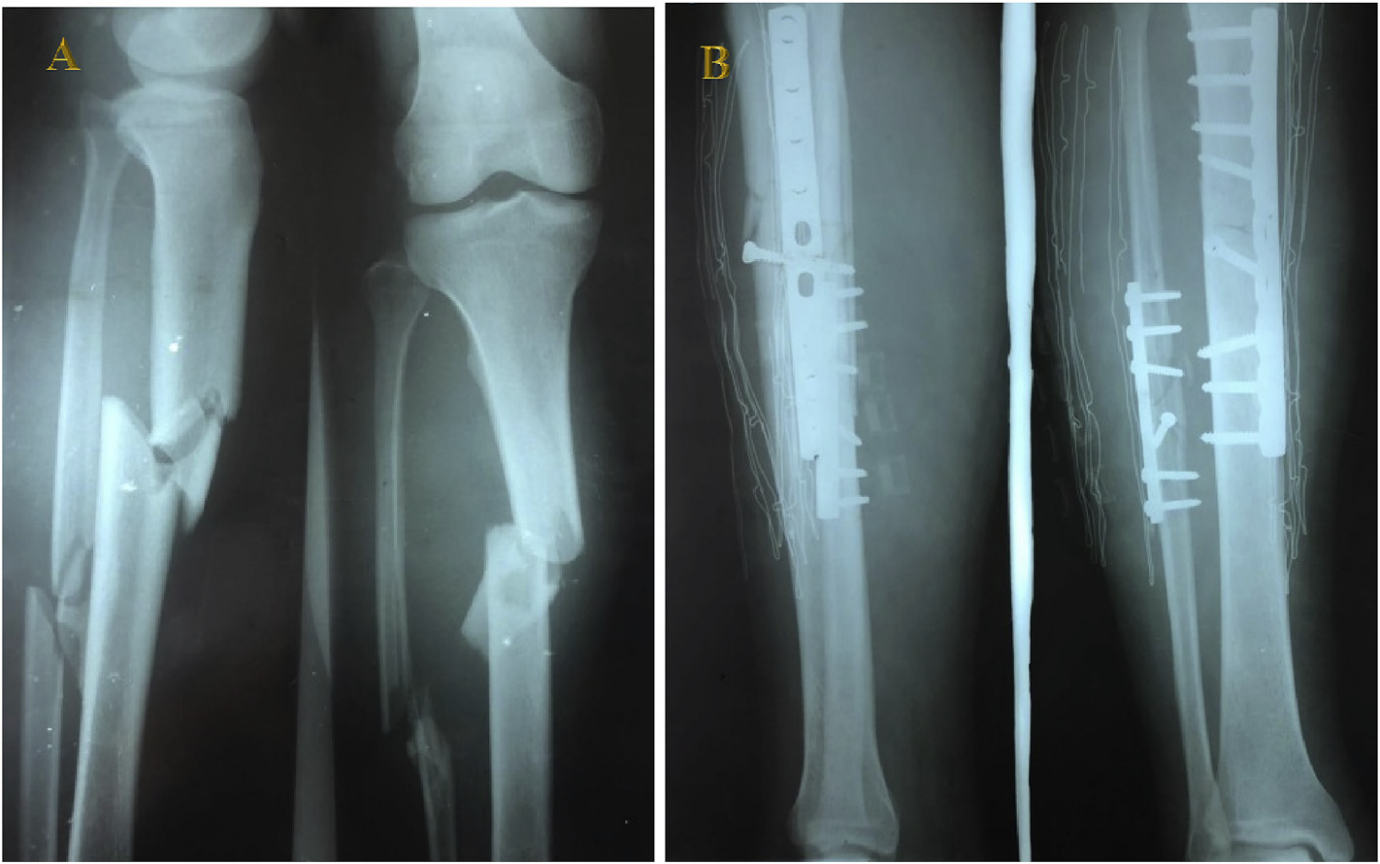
(A) Preoperative AP and lateral X-rays of the right leg showing a fracture of the tibia and fibula. (B) X-ray image of right-leg AP and lateral view showing internal fixation of the fracture.
